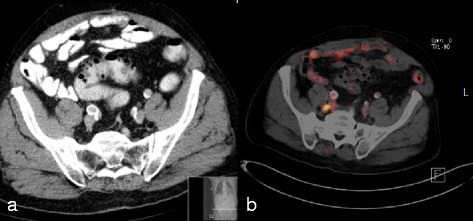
Up-staging due to lymph node involvement by ^68^Ga-PSMA-PET. Non-suspicious lymph nodes on MRI (a) presacral. In ^68^Ga-PSMA-PET imaging (b), the lymph node displays enhancement and was thus treated with a higher radiation dose
